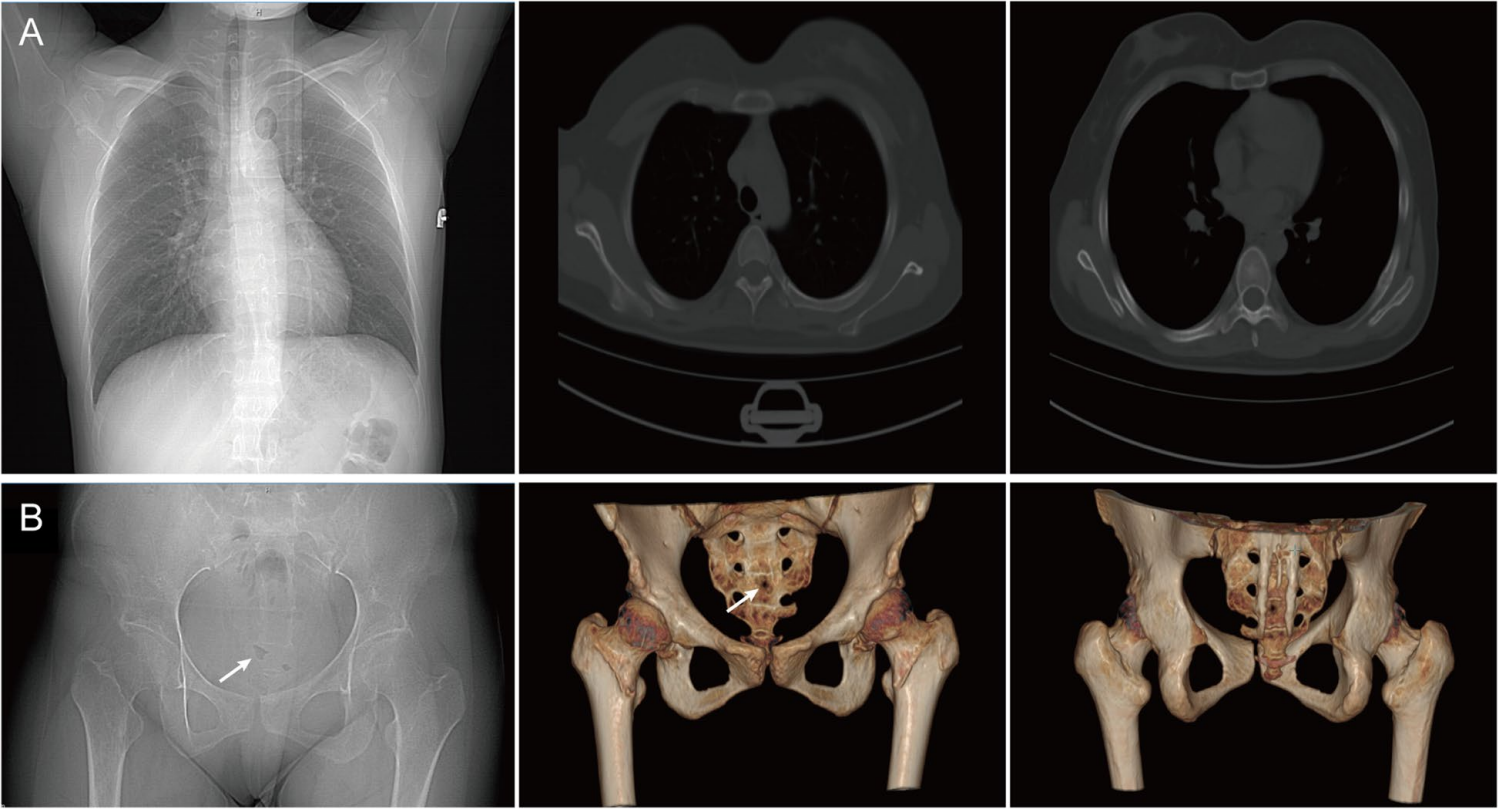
CT imaging of this patient. A Chest CT shows bone structure disorder in multiple ribs on both sides. (B) Hip joint CT shows nodular high density in the left ilium and sacrum, the bone structure of the remaining two hip joints is intact
